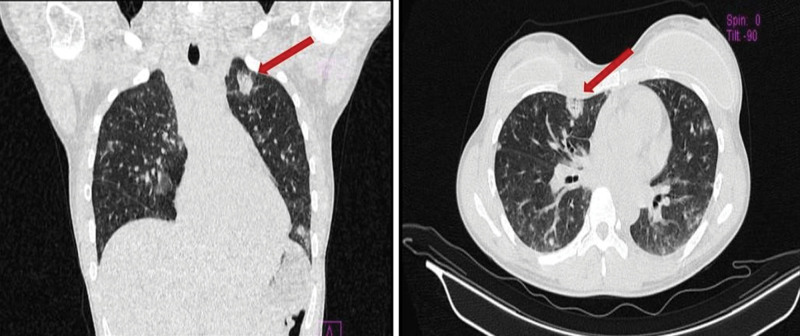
A non-contrast thoracic CT scan showed multiple bilateral nodules (red arrows) on admission.
